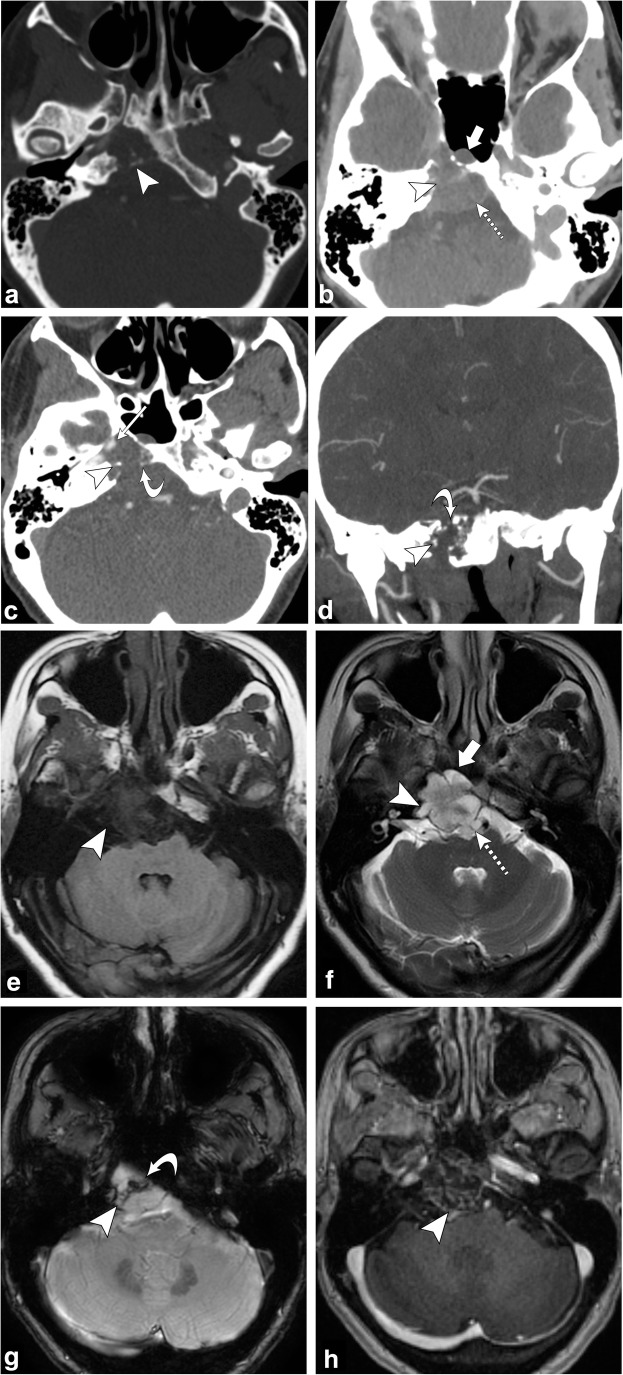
Chondrosarcoma. Axial non-contrast CT (a) shows an osteolytic destructive lesion involving the right petro-occipital junction and clivus (*arrowhead*). Axial contrast-enhanced CT (b) demonstrates this lesion to have a soft-tissue component extending anteriorly into the sphenoid sinus (*thick arrow*) and posteriorly into the prepontine cistern with mass effect on the pons (*dashed arrow*). Axial (c) and coronal (d) CT angiogram images demonstrate involvement of the right petrous carotid canal (*arrowheads*) with the lesion surrounding the right internal carotid artery (*thin arrow*). Note the punctate calcifications (chondroid matrix) within the mass (*curved arrow*). Axial T1-weighted (e) and axial T2-weighted (f) images show a lobulated lesion involving the right petro-occipital junction and clivus (*arrowhead*). Note the extension of the lesion anteriorly into the sphenoid sinus (*thick arrow*) and posteriorly into the prepontine cistern with mass effect on the pons (*dashed arrow*). Axial SWAN (g) shows internal foci of low signal, consistent with calcifications (*curved arrow*). Post-contrast axial T1-weighted imaging (h) demonstrates heterogeneous (“whorls” of) enhancement (*arrowhead*)
